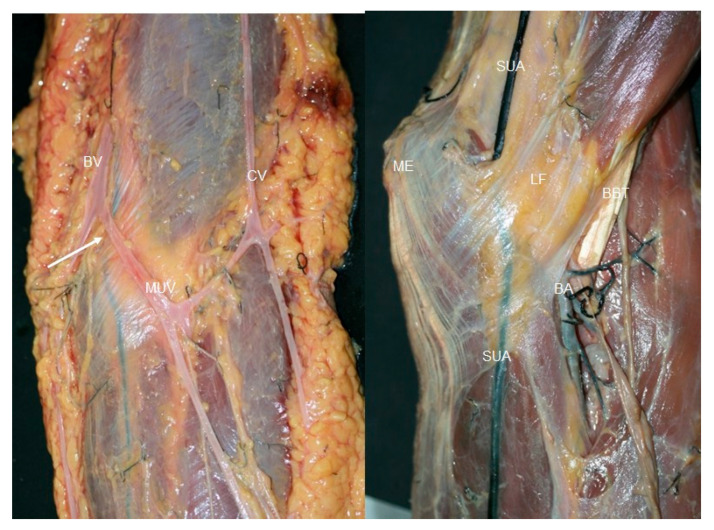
(Left) The anatomic dissection shows that before the basilic vein (BV), cephalic vein (CV) superficial ulnar artery (SUV),and white arrow crossed the elbow, it was crossed by the median ulnar vein (MUV), (right) and then ran deep to the bicipital aponeurosis (BA) surrounded by adipose tissue. Other structures that can be observed: superficial ulnar artery (SUA), medial epicondyle (ME), brachial artery (BA) and fascia of acertus fibrosus (LF).
